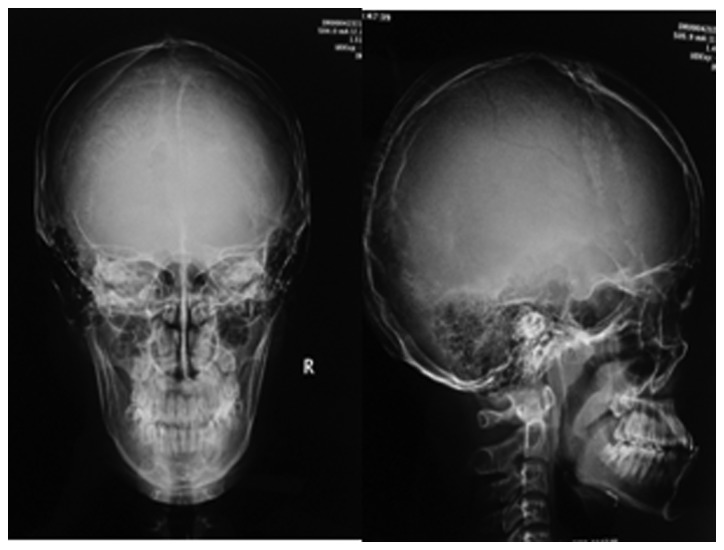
Lateral and frontal X-ray of the skull showed diffuse low bone density.
